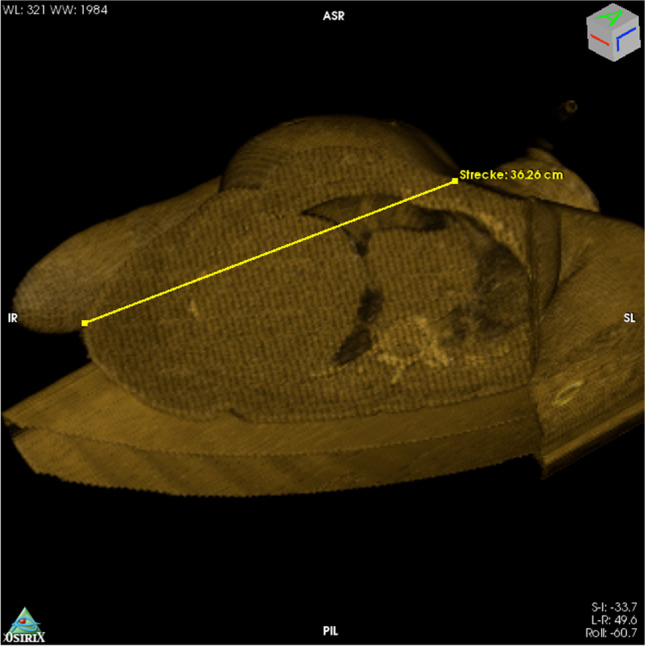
3D reconstructed CT datasets in volume-rendering mode, depicting the virtual wound trajectory and measurement of the straight connection between entry and exit wounds. After aligning the torso in the frontal plane, the cutting tool in OsiriX was used to cut the image along the bullet track. The parts of the torso that faced towards the lower left were eliminated. The ensuing cross section was then aligned in the frontal plane, and the exit and entry wounds were connected, using a measuring tool. The approximately 36-cm-long bullet trajectory can be seen to pass through the thoracic and the abdominal cavities
